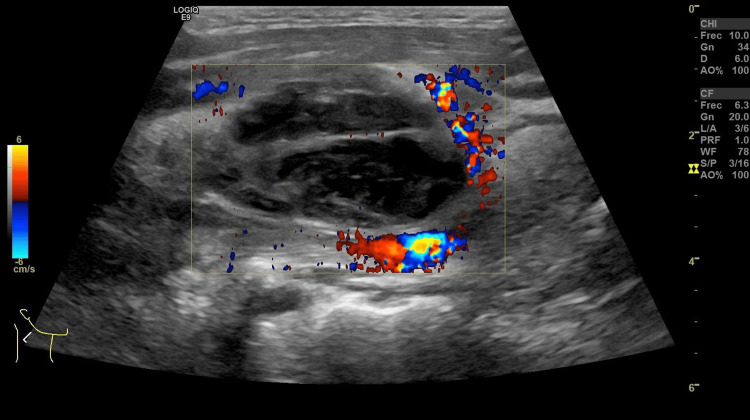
The initial neck ultrasound shows a heterogeneous, predominantly hypoechoic, non-vascularized, septated lesion compatible with Ludwig’s angina.
